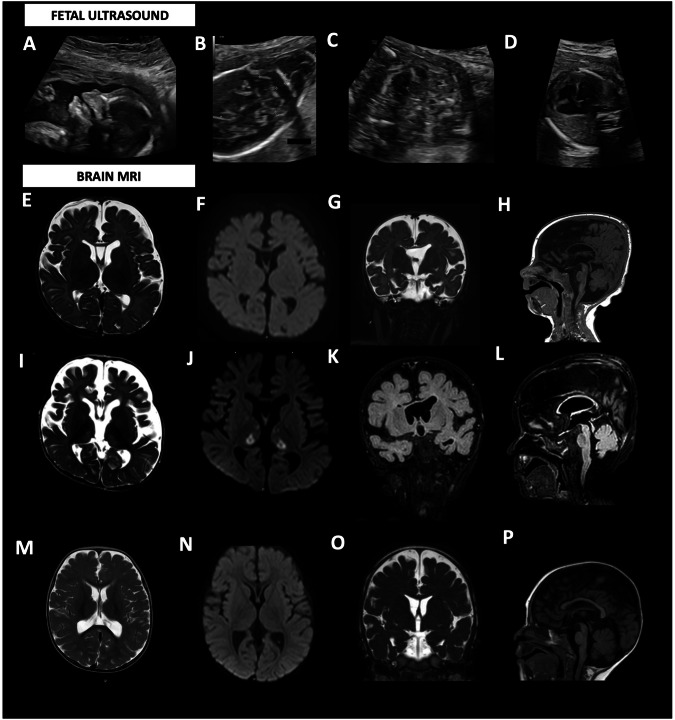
Imaging studies. A–D Ultrasound images at 20 weeks of gestation in P1.a showing dysmorphic profile (A), cystic hygroma (17.5 mm) (B), and echogenic bowel with a mildly dilated loop (C); and at 28 weeks gestation in P1.b showing cardiac biventricular hypertrophy, accompanied by diminished contractility in both the right and left ventricles (D). Patient 1.a Brain MRI at 4 months: (E) axial section T2-weighted, (F) axial section DWI (b = 1000), (G) coronal section T2-weighted, (H) sagittal T1-weighted section. The images show brainstem hypoplasia and mega cisterna magna, severe thinning of the corpus callosum, and moderate enlargement of the ventricular system and subarachnoid spaces. I–L Patient 1.a Brain MRI at 8 months: (I) axial section T2-weighted, (J) axial section DWI (b = 1000), (H) coronal section FLAIR T2-weighted, (L) sagittal section FLAIR T2-weighted. The images showing in DWI restricted signal of both thalami, hyperintense in T2 weighted images, and progressive increase in subcortical cortical atrophy. Patient 1.b Brain MRI at 11 months: (M) axial section T2-weighted image, (N) axial section DWI (b = 1000), (O) coronal section T2-weighted, (P) sagittal section T1-weighted section. The images show mild thinning of the corpus callosum, mild hypoplasia of the brainstem, and moderately wide cisterna magna, ventricular system, and subarachnoid spaces.
